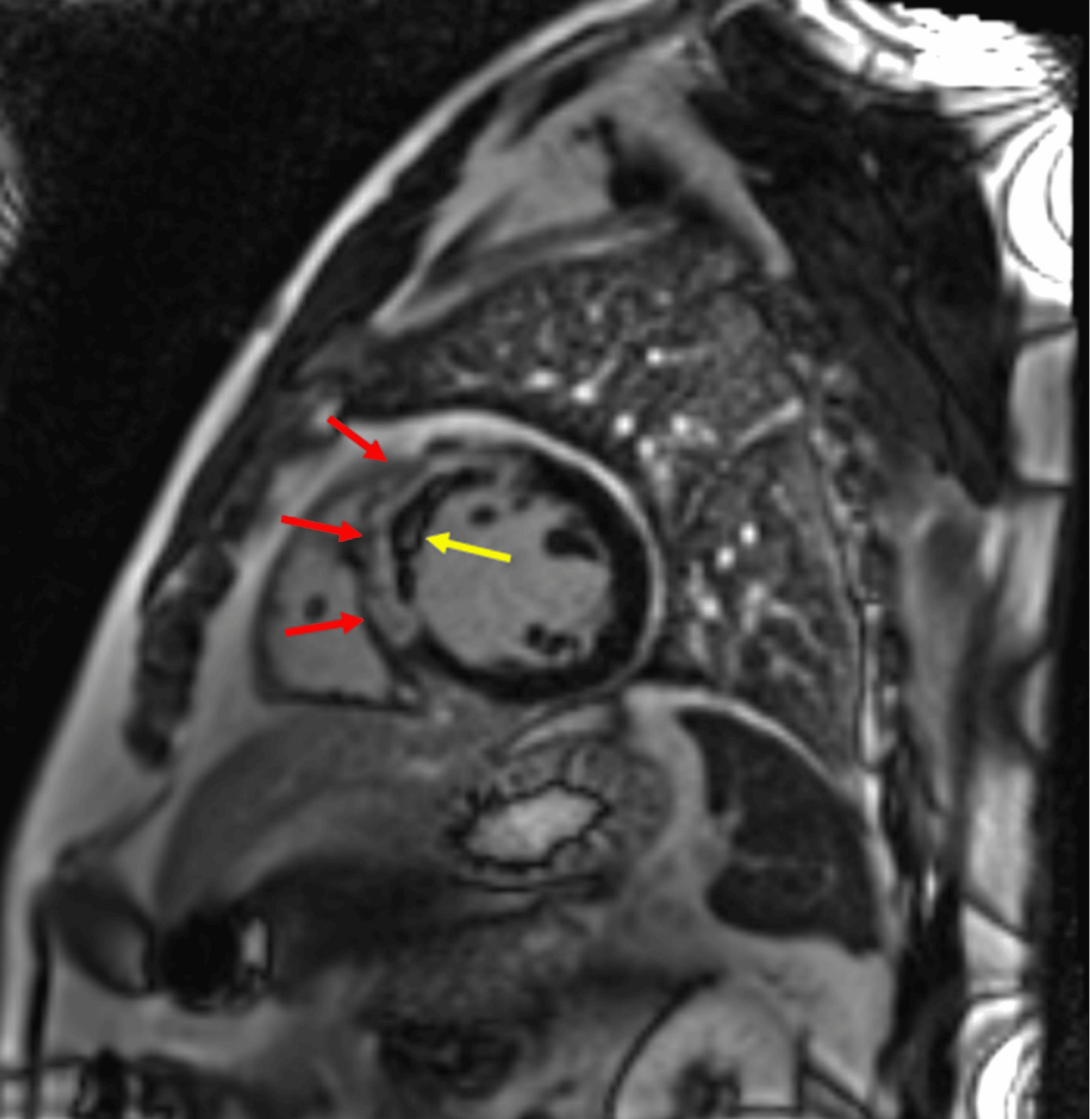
Sagittal view of cardiac MRI on the left showing late gadolinium enhancement (red arrows) with black core (yellow arrow) in the anteroseptal wall of the left ventricle suggestive of myocardial ischemia with microvascular occlusion.
